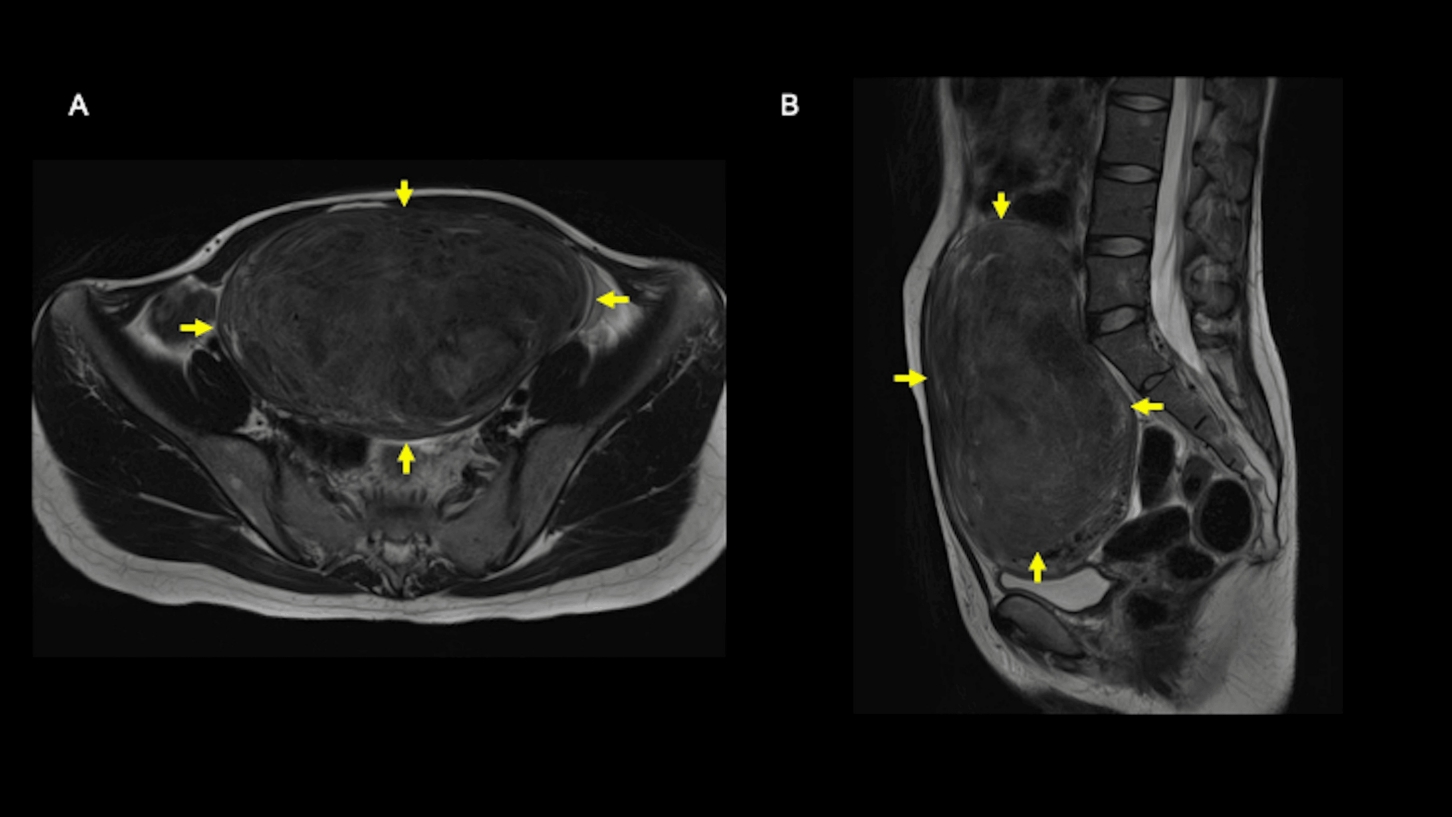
T2-weighted magnetic resonance imaging shown in the axial (A) and sagittal (B) views A 16-cm pelvic mass (yellow arrow) of unknown location in relation to the uterus and ovaries was detected.
